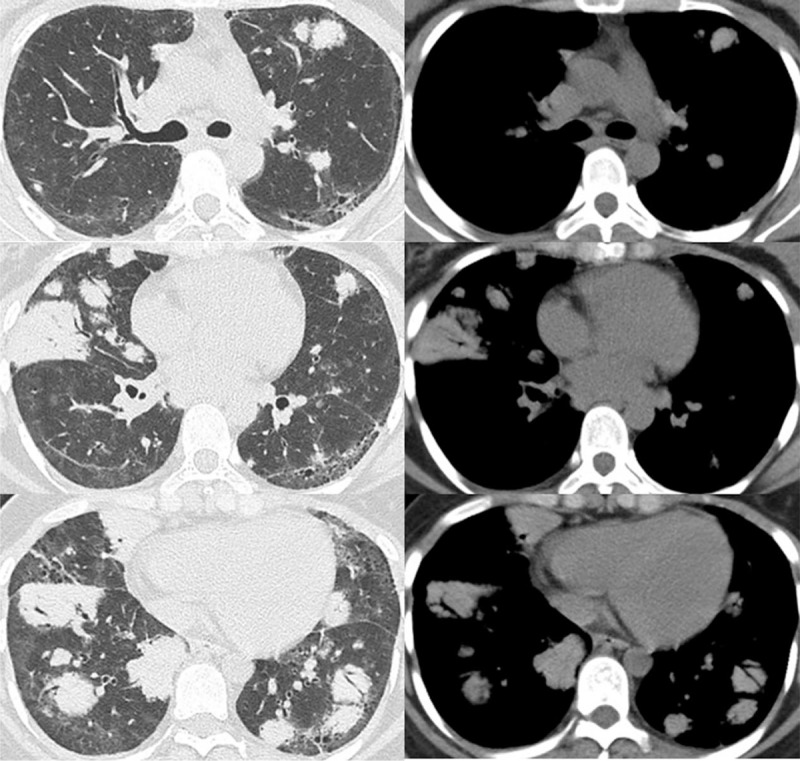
Chest CT showing multiple bilateral lung nodules. CT = computed tomography.
